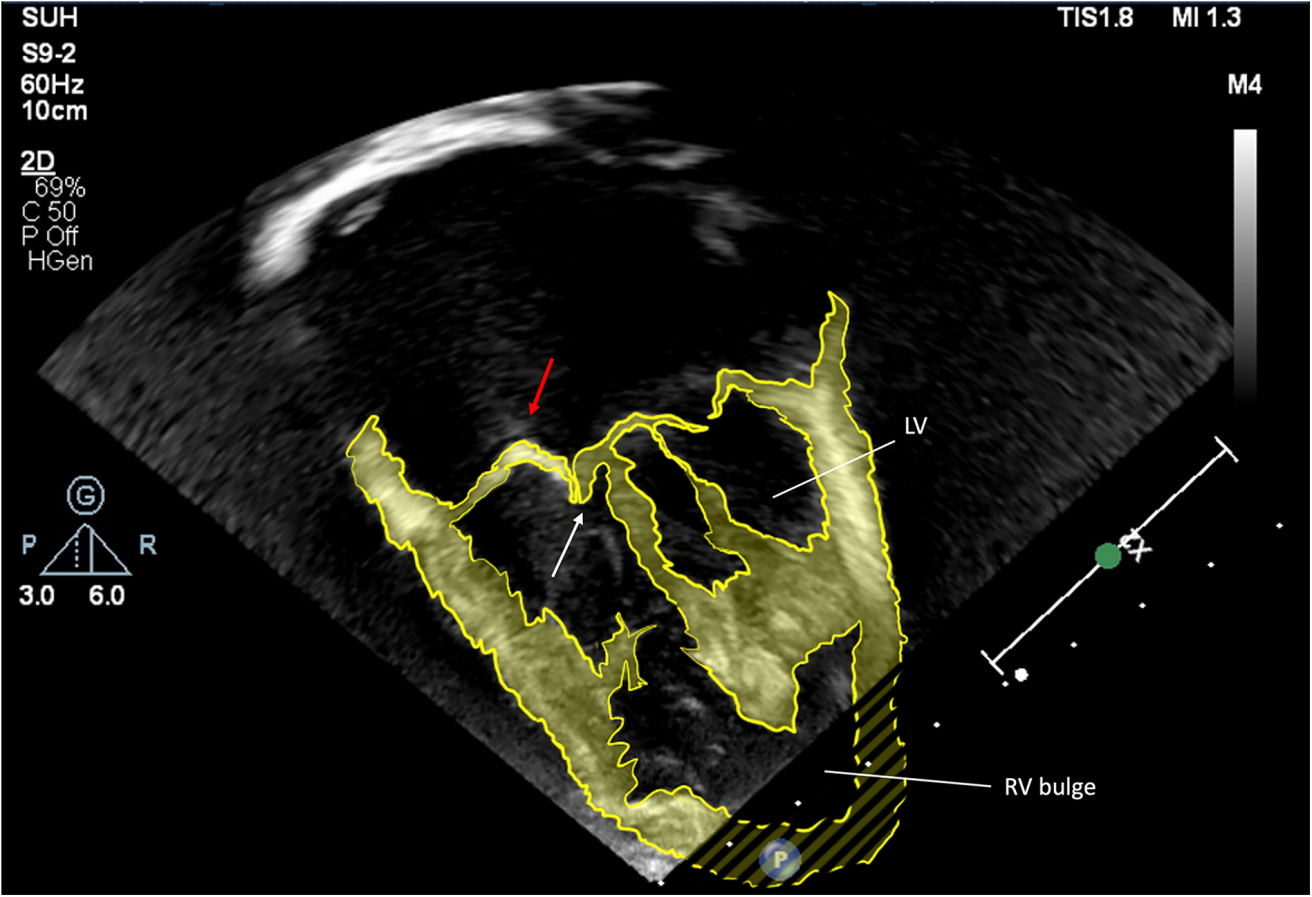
Apical view, enhanced 2D echocardiographic image. Antero-superior leaflet prolapse (red arrow), combined with tethering of the septal leaflet (white arrow). The right ventricular bulge is indicated.
